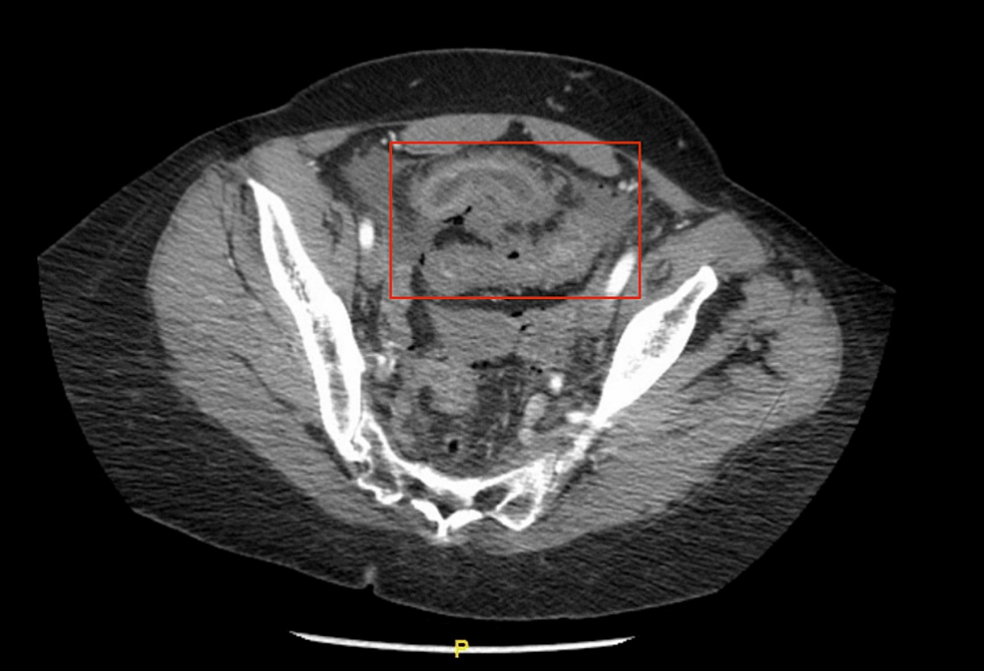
CT Angiography/Abdomen and Pelvis With and Without Contrast Axial View The abdomen and pelvis CT scan revealed the presence of free air and a fistula between the small bowel and the sigmoid colon (red box).
